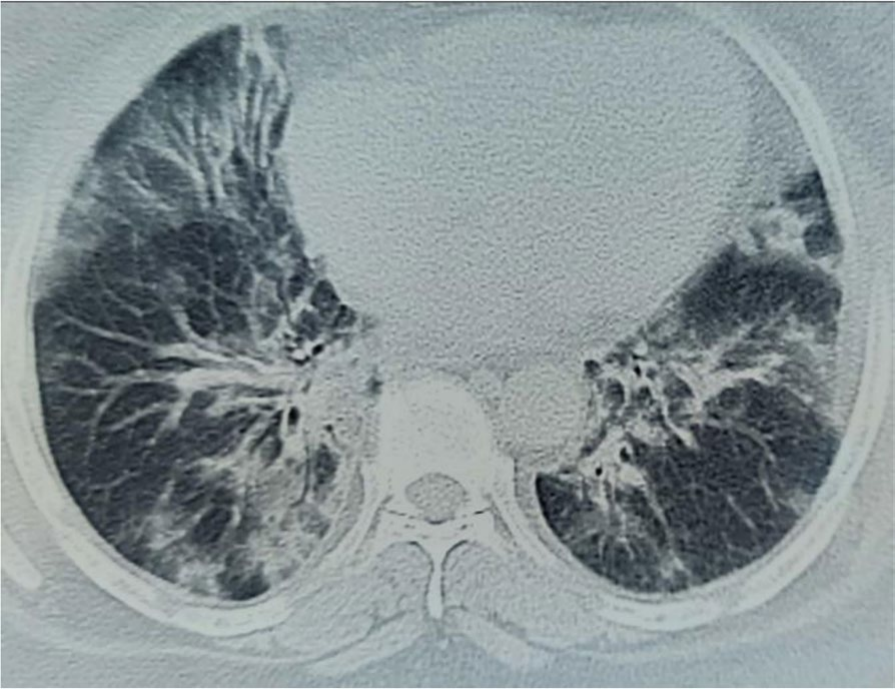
HRCT done on presentation to the ER that shows B/L ground glass infiltrates with patchy consolidations involving mainly the peripheries
